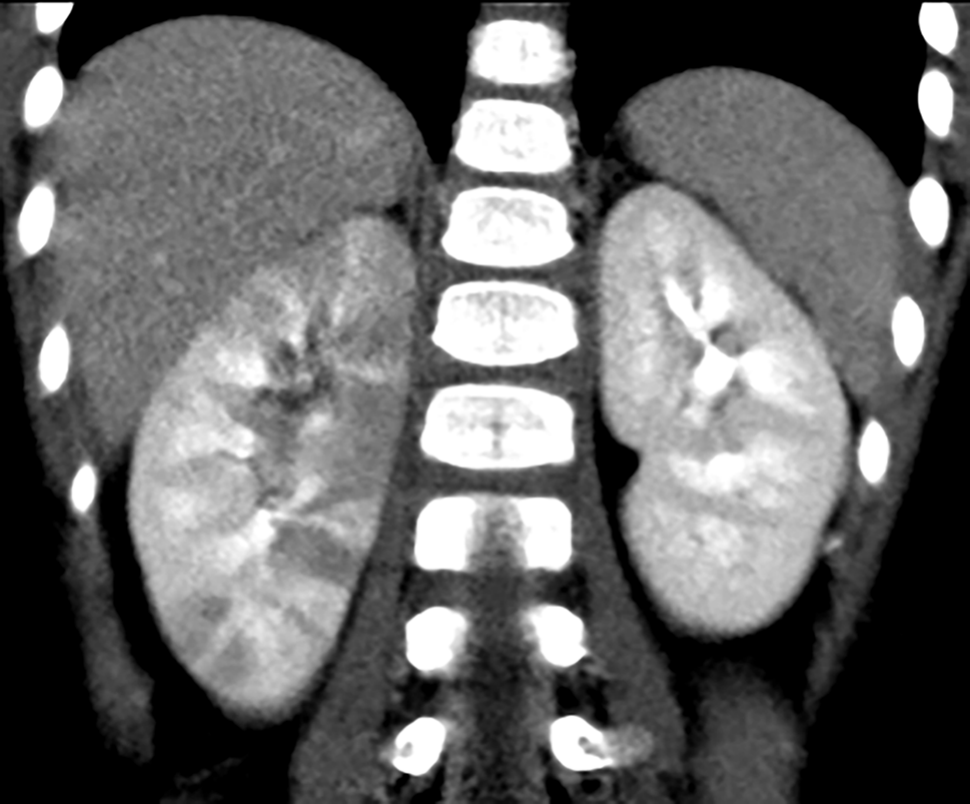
A 3 year-old female presented with fever, cough, vomiting, diarrhea, and leukocytosis (14,900 WBC/mL). Urinalysis and culture obtained from bladder catheterization were negative. A CT abdomen was obtained, due to concern for appendicitis, that demonstrated right-sided pyelonephritis. Coronal post-contrast CT demonstrates multifocal wedge-shaped hypodensities with striated nephrogram, and diffuse swelling of the right kidney
